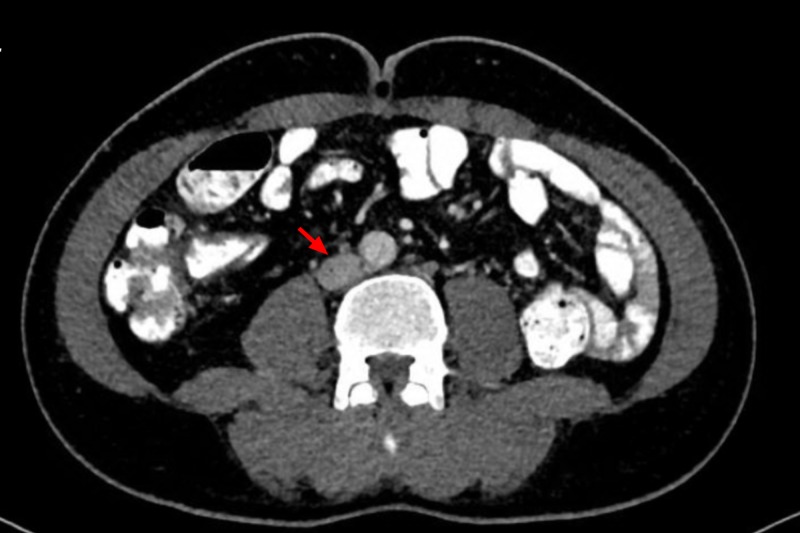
Computed tomography (CT) scan of the abdomen Red arrows: para-aortic lymph node.
